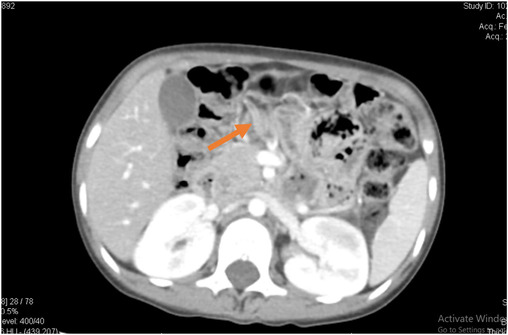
CT abdomen of the patient (axial view).
